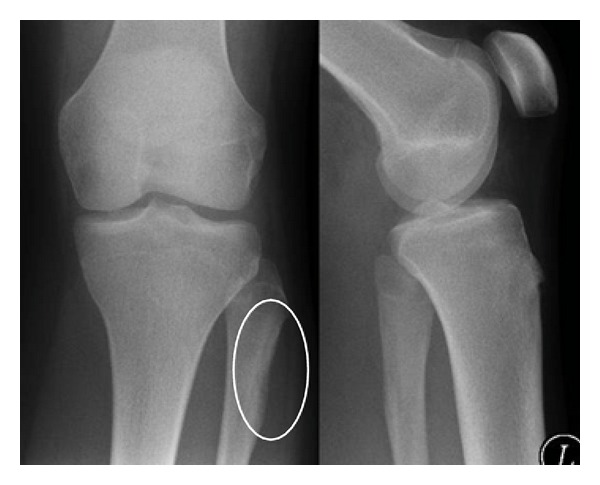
Preoperative X-ray showing local lucent nidus at the left proximal fibula.
